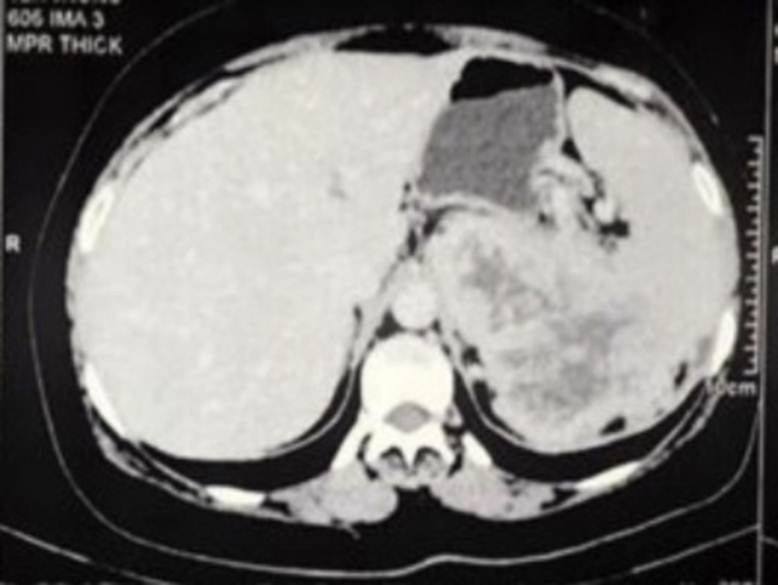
Axial CT abdomen showing large left renal mass infiltrating the spleen and pancreas.
